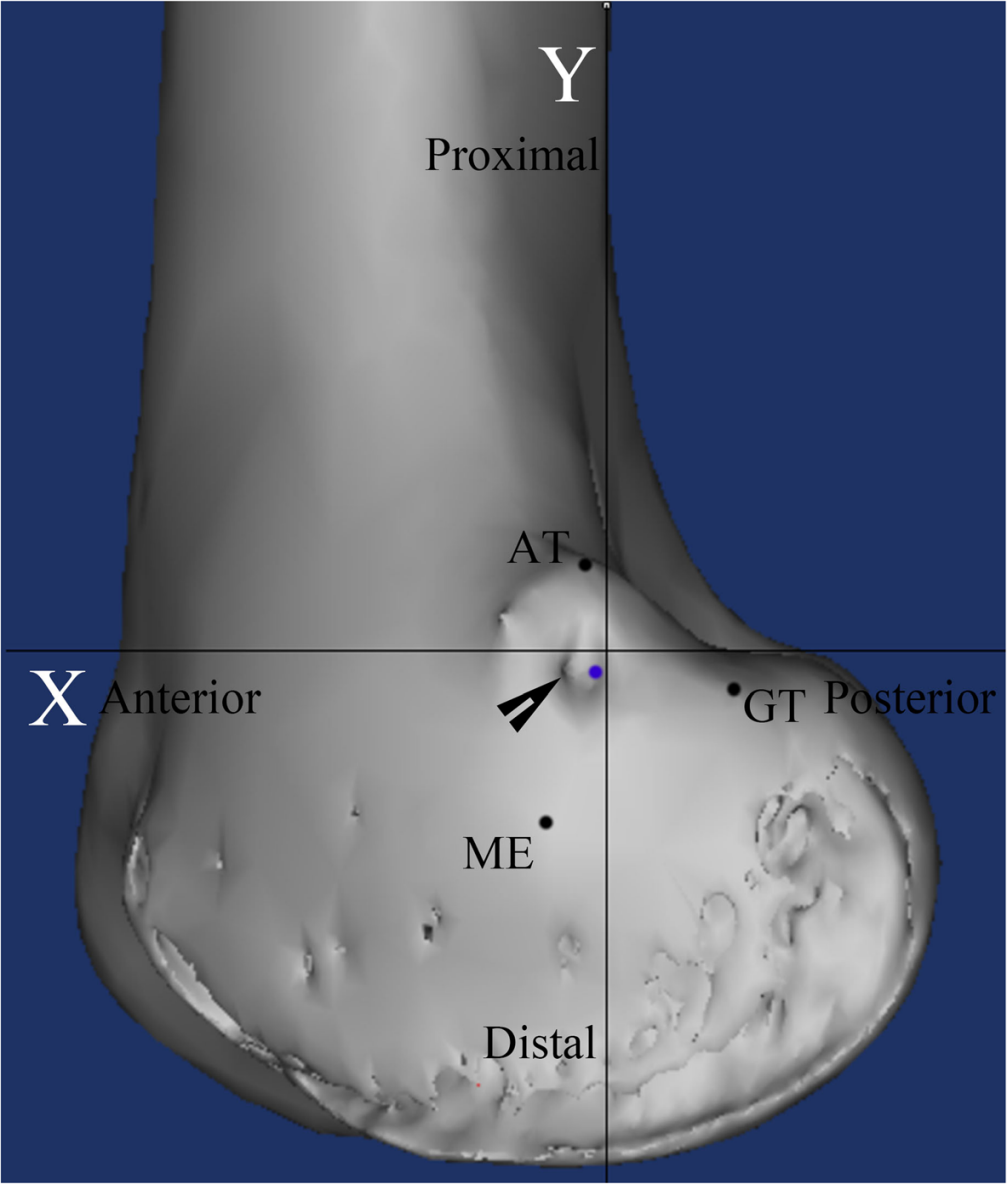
A representative computed tomography image showing the assessment of the accuracy of the medial patellofemoral ligament femoral tunnel. The blue circle indicates Schöttle point, and the black arrow indicates the center of the medial patellofemoral ligament femoral tunnel established using the sulcus localization method. AT: adductor tubercle; ME: medial epicondyle; GT: gastrocnemius tubercle
